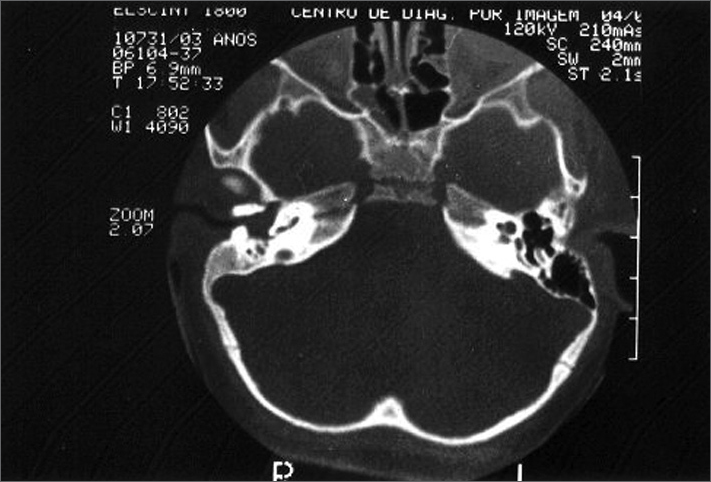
Axial view of a temporal bone CT scan: tapering external ear canal atresia, not reaching what would be the tympanic membranes. No mastoid antrum cell aeration and tympanic cavity filled by soft tissue in the right ear.
